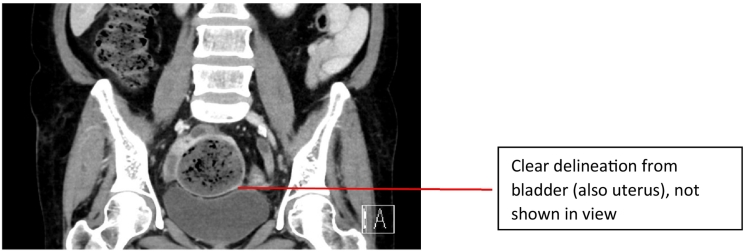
Coronal view of CT scan at presentation.
